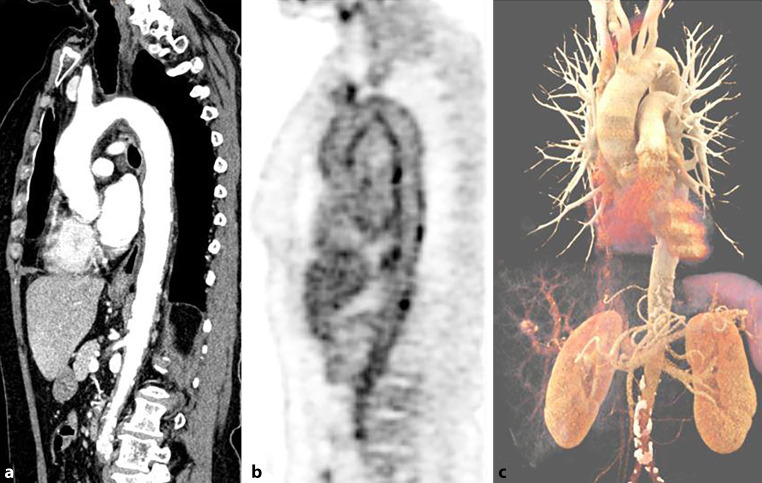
[^18^F]FDG PET/CT image showing an active aortitis with vessel wall thickening in sagittal reformatted CT angiography images (a) and [^18^F]FDG uptake at the aortic arch and the descending aorta (b). 3D volume rendering of the aorta shows no pathologic dilatation of the vessel (c). The tracer activity of 263MBq corresponding to 7.1 mSv and a DLP of 1625 mGy*cm corresponded to 24.375 mSv, and made up a total effective dose of 31.5 mSv
